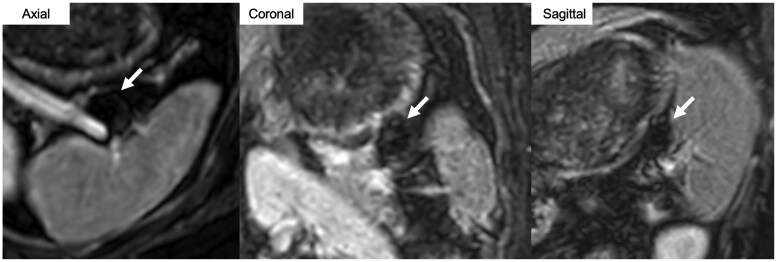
Contrast-enhanced magnetic resonance image after splenic aneurysm embolisation. Contrast-enhanced magnetic resonance imaging performed 2 months after coil embolisation shows complete occlusion of the splenic aneurysm with no evidence of coil compaction, aneurysm recurrence, and splenic infarction.
